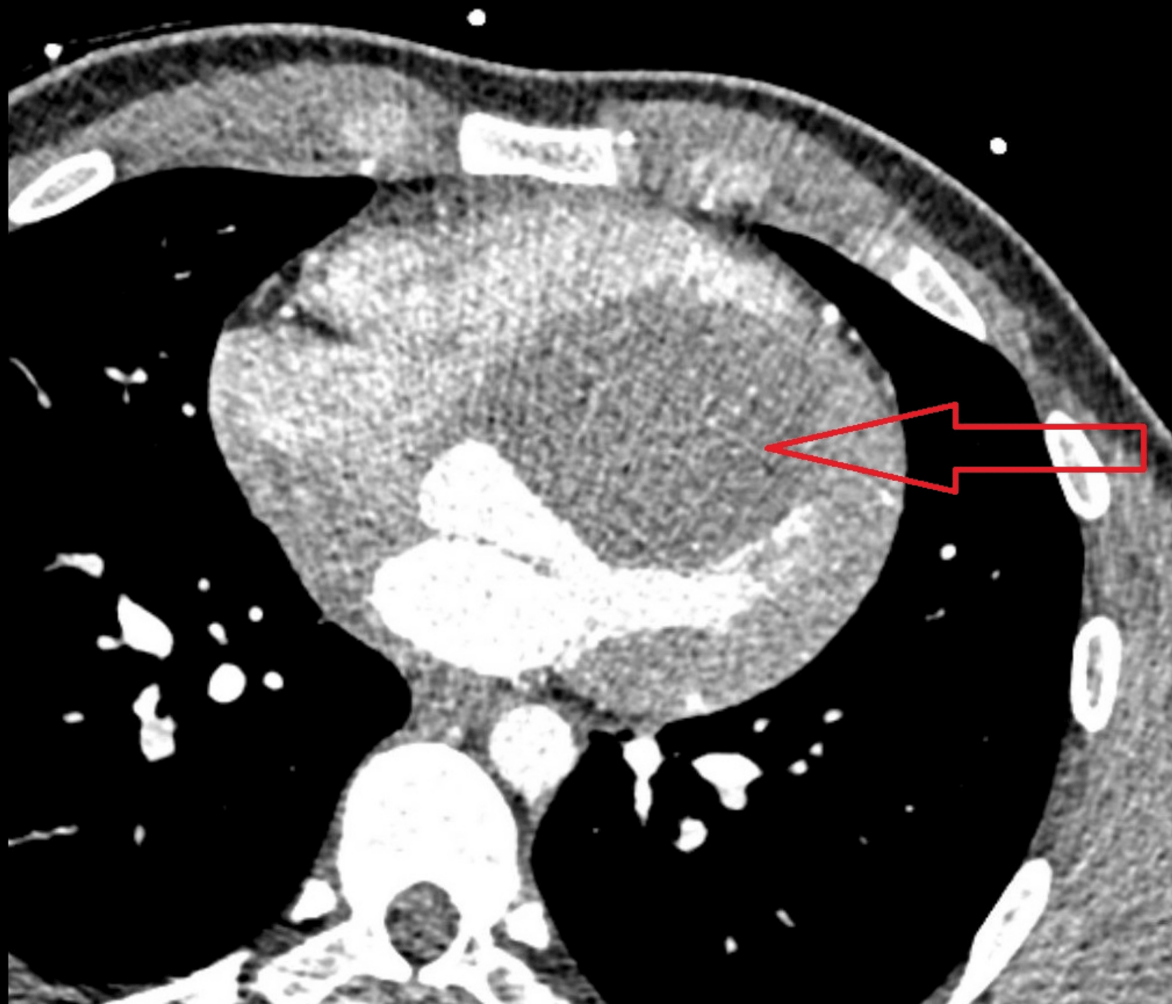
Coronary computed tomography angiography (axial view) showing a large fibroma causing LVOT obstruction LVOT, left ventricular outflow tract
